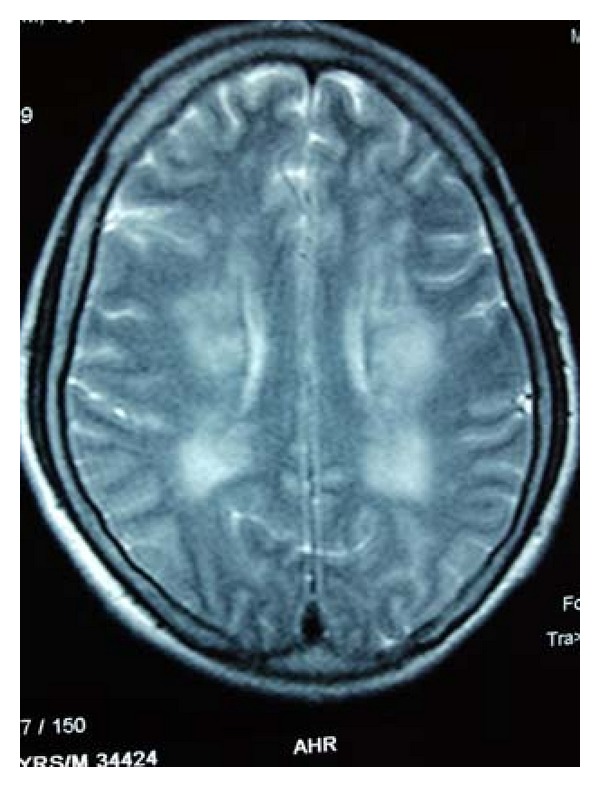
MRI Brain showed nonspecific ischemic changes.
